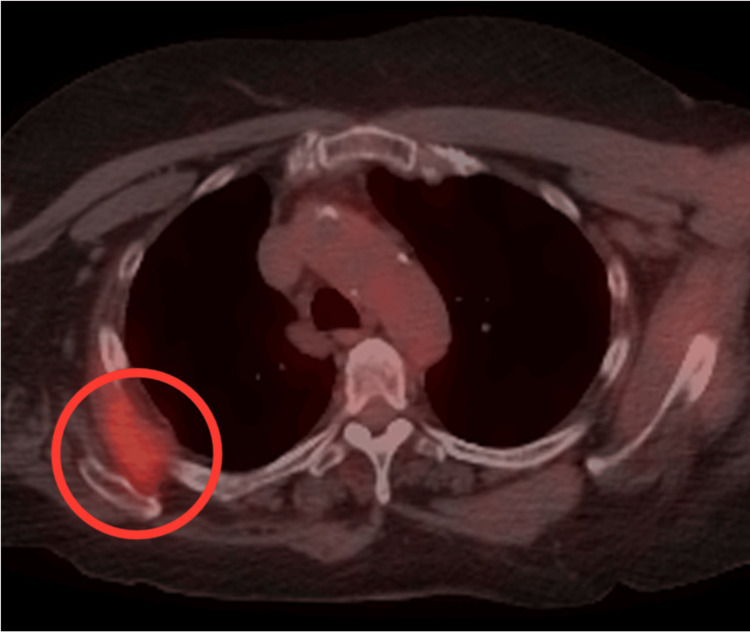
Chest PET-CT showing soft tissue uptake in the periphery of the right upper posterior chest wall (red circle), between the lower angle of the scapula and the subjacent ribs, with a maximum SUV of 3.9. PET-CT: positron emission tomography-computed tomography scan; SUV: standardized uptake value
